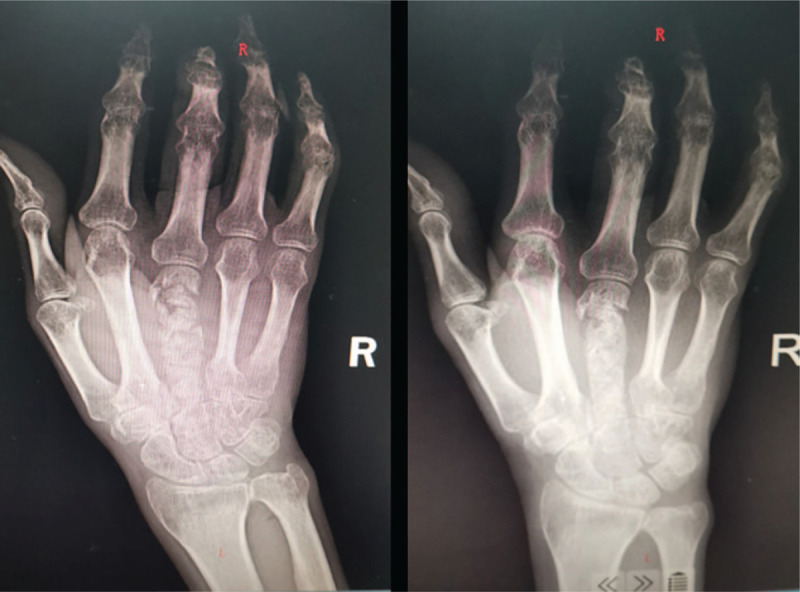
X-ray image during follow-up. left (1 mo), right (3 mo).
